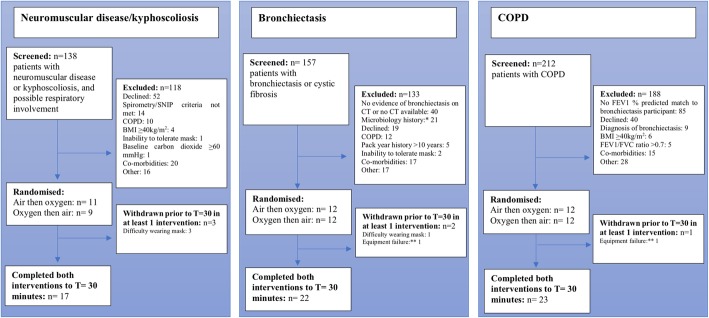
Flow of participants through studies. BMI: Body mass index, COPD: Chronic obstructive pulmonary disease, CT: Computerised tomography, FEV_1_: Forced expiratory volume in 1 s, FVC: Forced vital capacity, SNIP: Sniff nasal inspiratory pressure. *Burkholderia or other result at the investigator’s discretion.  T valve tubing malfunction for 1 participant in the Bronchiectasis study and Sentec maintenance error for 1 participant in the COPD study. See Online Supplement for further detail. See legend of Table 3 and Online Supplement for further N value details
